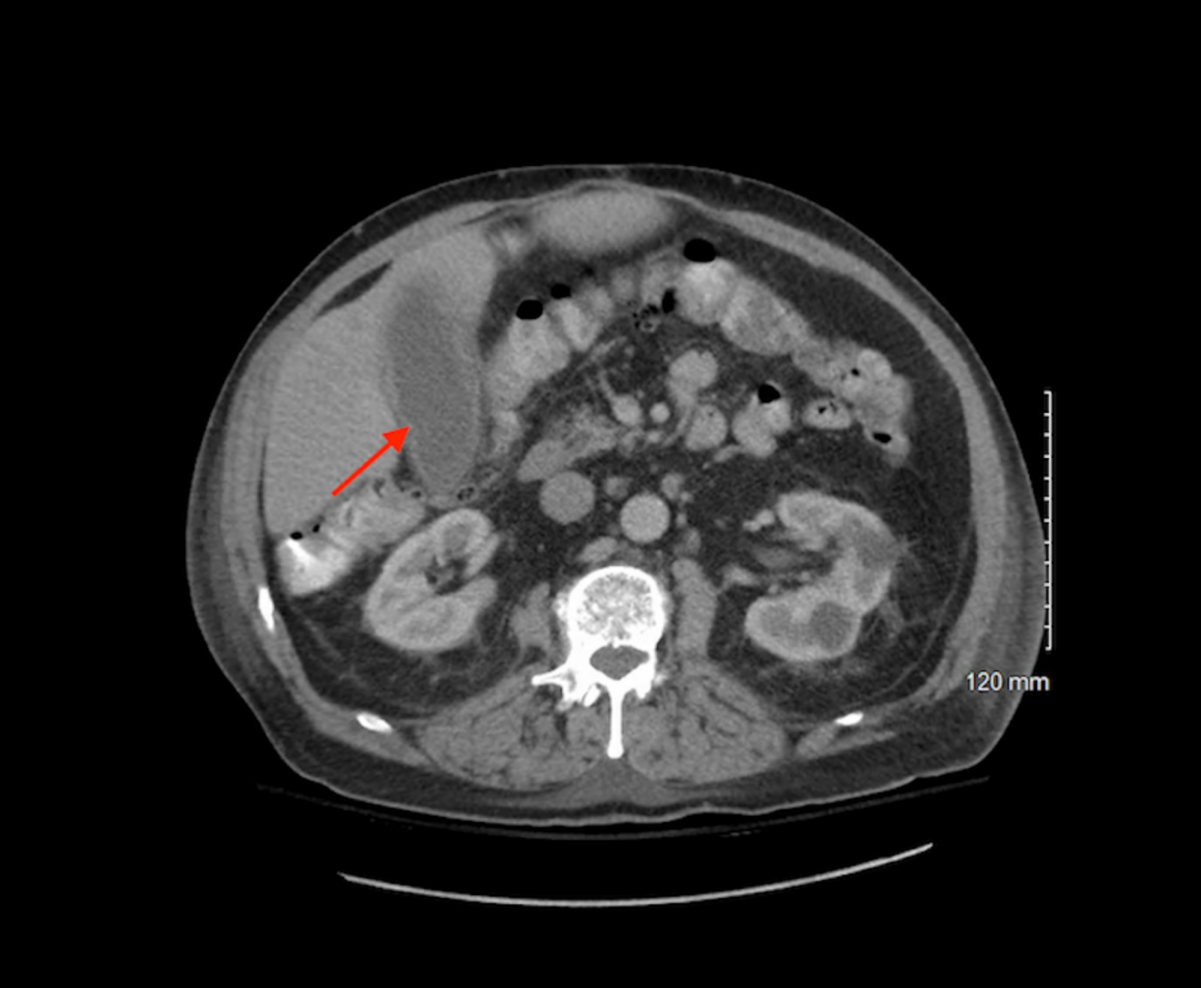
Computed tomography of the abdomen showing dilated gallbladder.
